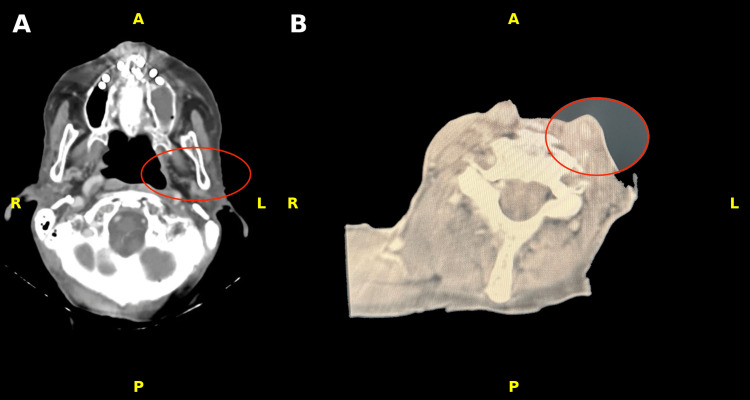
Follow-up CT of the neck (June 2025) demonstrating a maintained complete response (A) Axial section at the level of the prior region of the primary lesion. (B) Axial section at the level of the previous recurrent cervical lesion. In both panels, the red ellipse delineates the site of the original lesion. No residual soft-tissue mass, anomalous contrast enhancement, or pathological lymphadenopathy is identified within these regions or bilaterally, confirming the absence of local or nodal recurrence. Postsurgical changes, tracheostomy, and cervical fibrosis are noted CT: computed tomography
